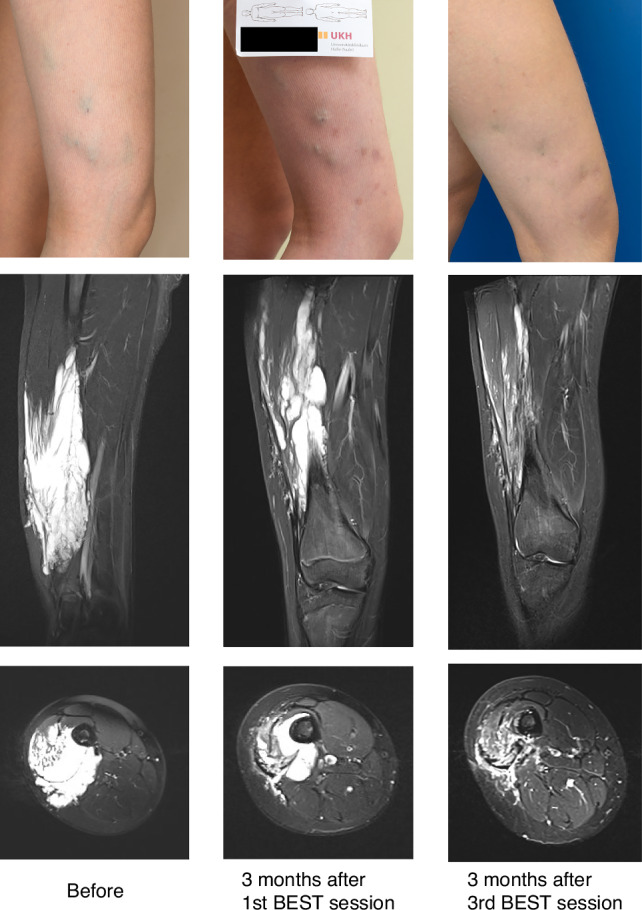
Female patient (13 years at start of therapy) with a venous malformation on her right thigh. Time series over 3 years with photographs and MR images (T2 STIR in coronal and transversal plane) obtained before therapy, 3 months after the 1st BEST session (at age 13) and 3 months after the 3rd BEST session (at age 15).
